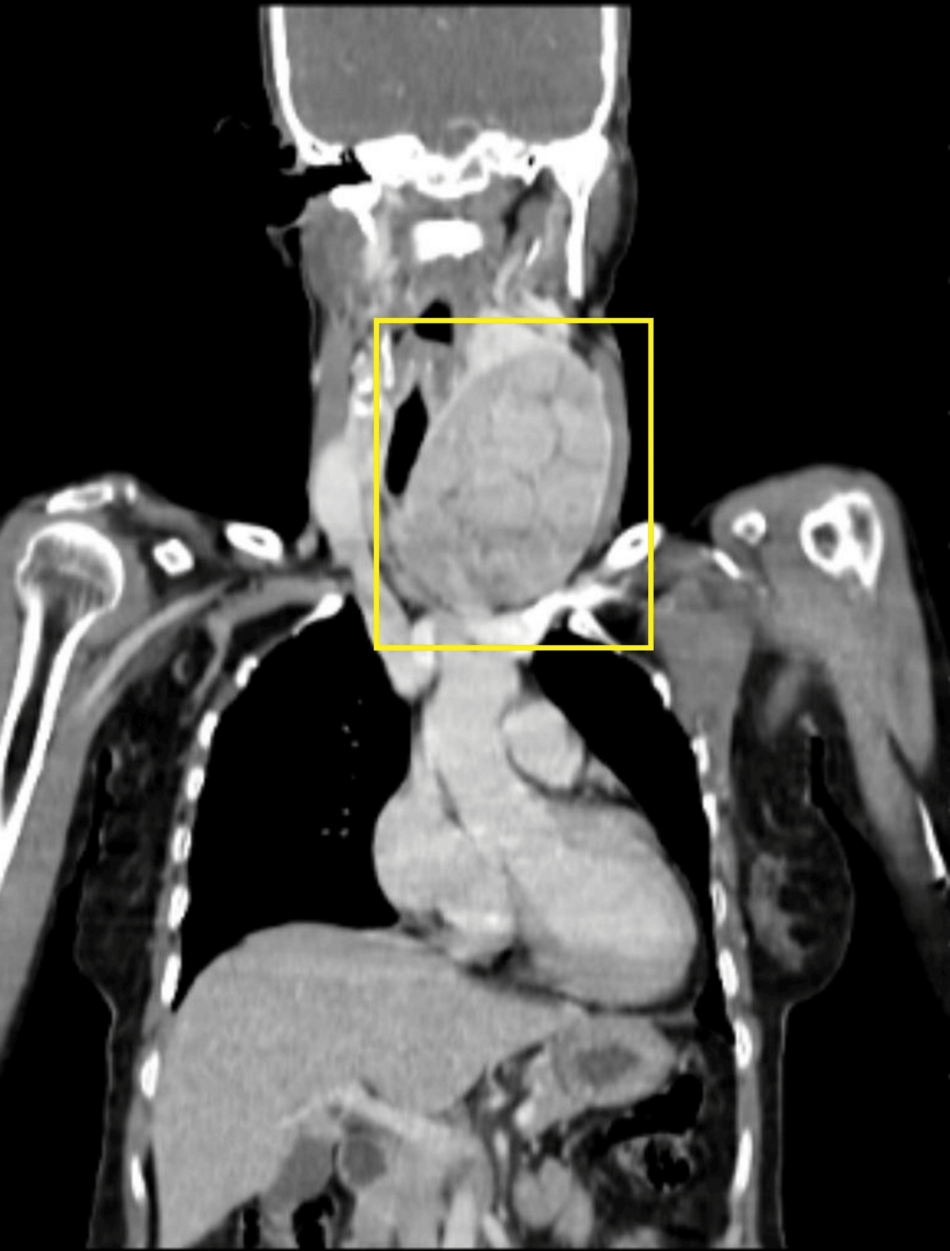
CECT showing the thyroid nodule (coronal view) showing an oval-shaped, well-circumscribed, multinodular soft tissue lesion that shows vivid enhancement CECT: contrast-enhanced computed tomography
